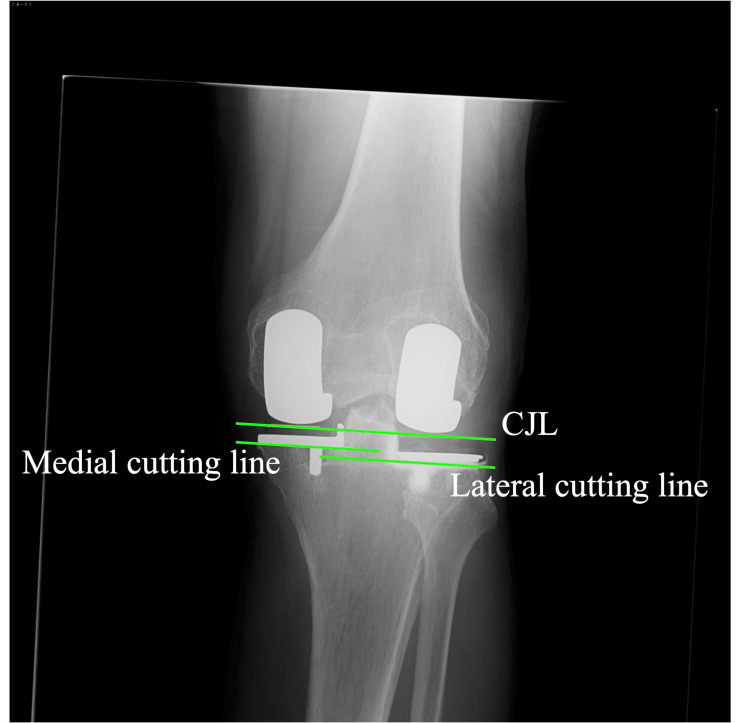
Postoperative radiography The coronal joint line (CJL) is parallel to the cutting lines. Note that the lateral cutting line is lower than the medial cutting line.
